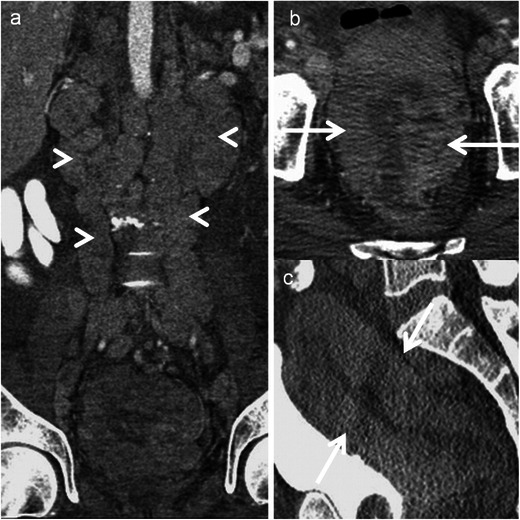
Mantle cell lymphoma in a 58-year-old man presenting with rectal bleeding and tenesmus.a Coronal, b axial and c sagittal contrast-enhanced CT reveal a long segment of marked circumferential wall thickening (*arrow*) involving the rectum with multiple polypoidal intraluminal projections. There is aneurismal dilatation of the rectum with extensive retroperitoneal lymphadenopathy (*arrowhead*)
